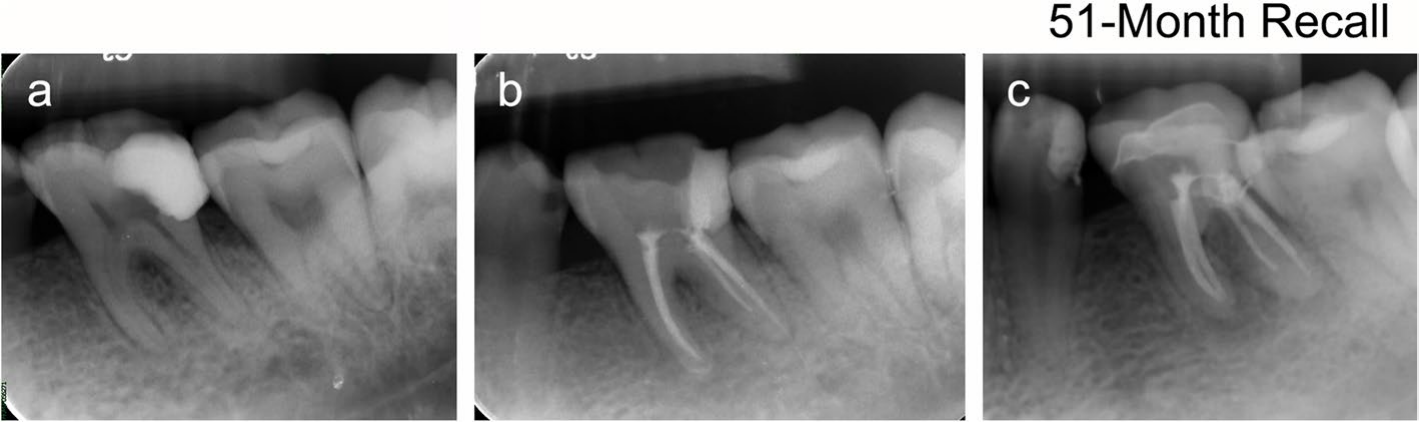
Preoperative (a), postoperative (b), and recall (c) radiographs of outcomes of unhealed teeth
